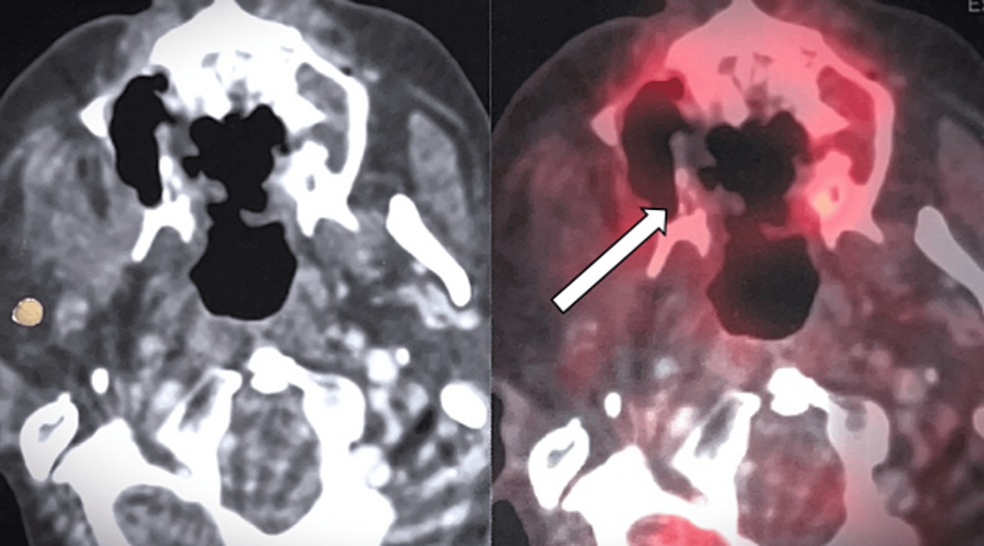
18F-FDG PET/CT scan with contrast. No evidence of FDG avid enhancing lesion seen in left side of hard palate with post-treatment defect noted (previously 13 mm × 10 mm × 15 mm, SUVmax: 7.2). No evidence of FDG avid-enhancing lesion seen in right side of hard palate, adjacent right upper alveolar region with post-treatment defect noted (previously 17 mm × 7 mm × 25 mm, SUVmax: 7.1). FDG: fluorodeoxyglucose, PET: positron emission tomography.
